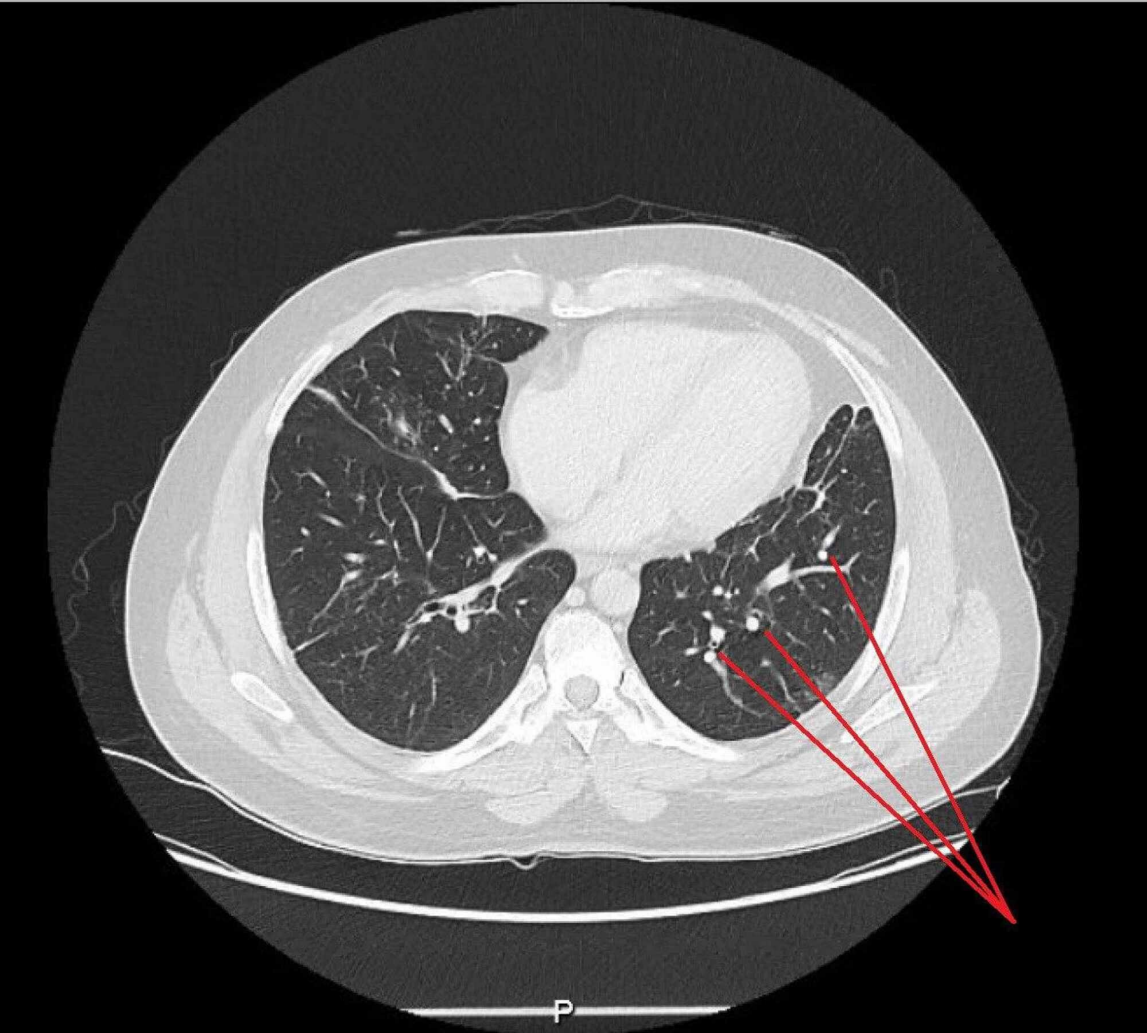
Computed tomography of the lungs showing micronodules
